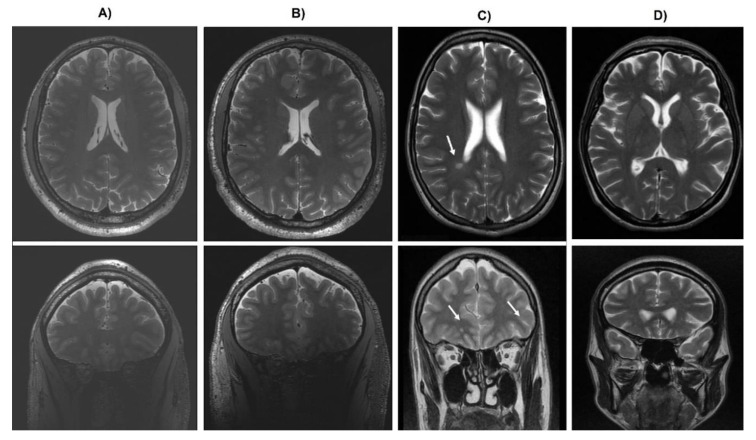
T2 Magnetic resonance images captured from (A) health participant (H), (B) participant with diagnosed schizophrenia (S), (C) patient with white matter hyperintensities (SN), (D) patient (N).
